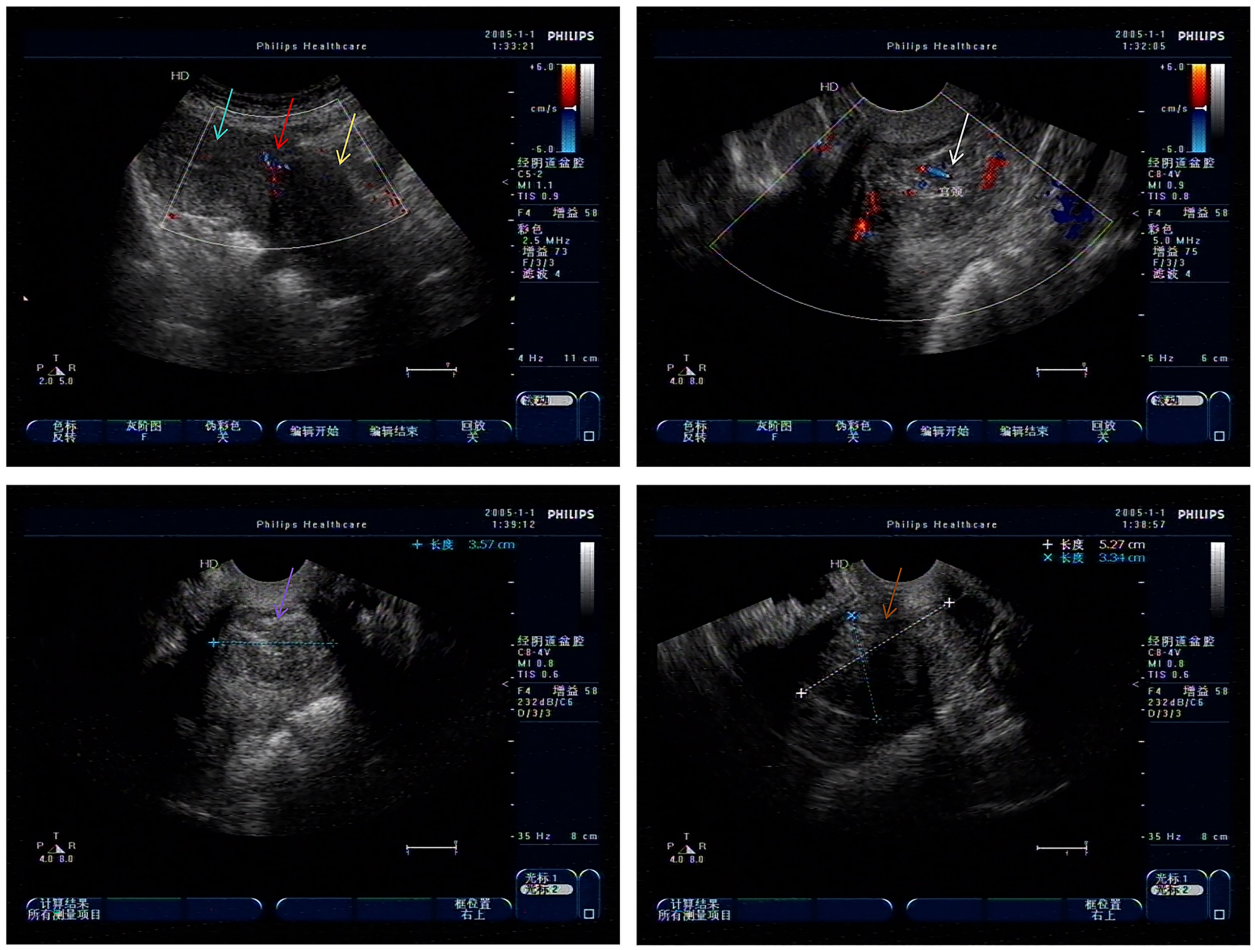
Low echo of cervical canal in gynecological ultrasound examination (Green arrow: uterine body; Red arrow: uterine scar; Yellow arrow: cervical area; White arrow: Cervical hypoechogenicity and blood flow; Purple and brown arrow: Cervical hypoechogenicity).
